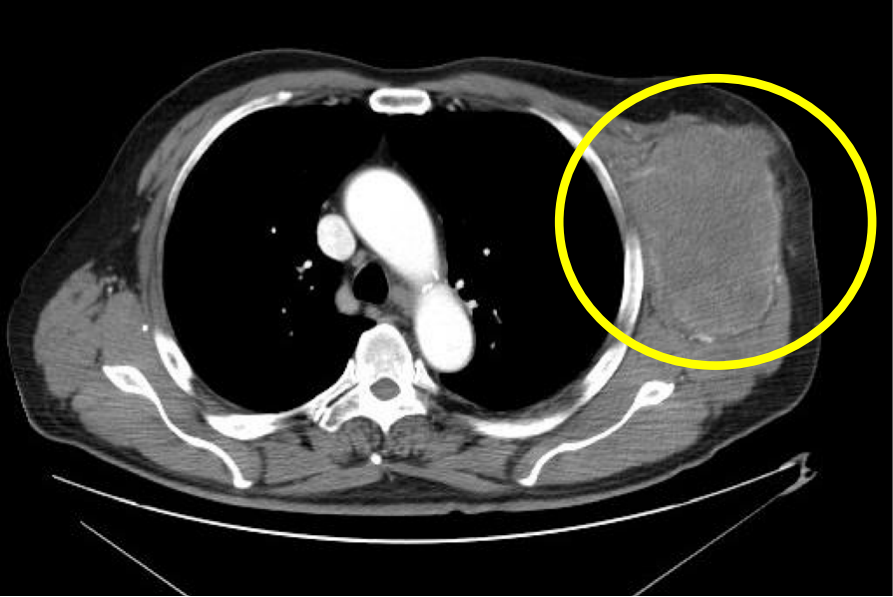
Contrast-enhanced chest computed tomography. The tumor in the left axilla measured 11 cm × 7 cm × 11 cm. It had a relatively distinct border, but its surface was irregular
